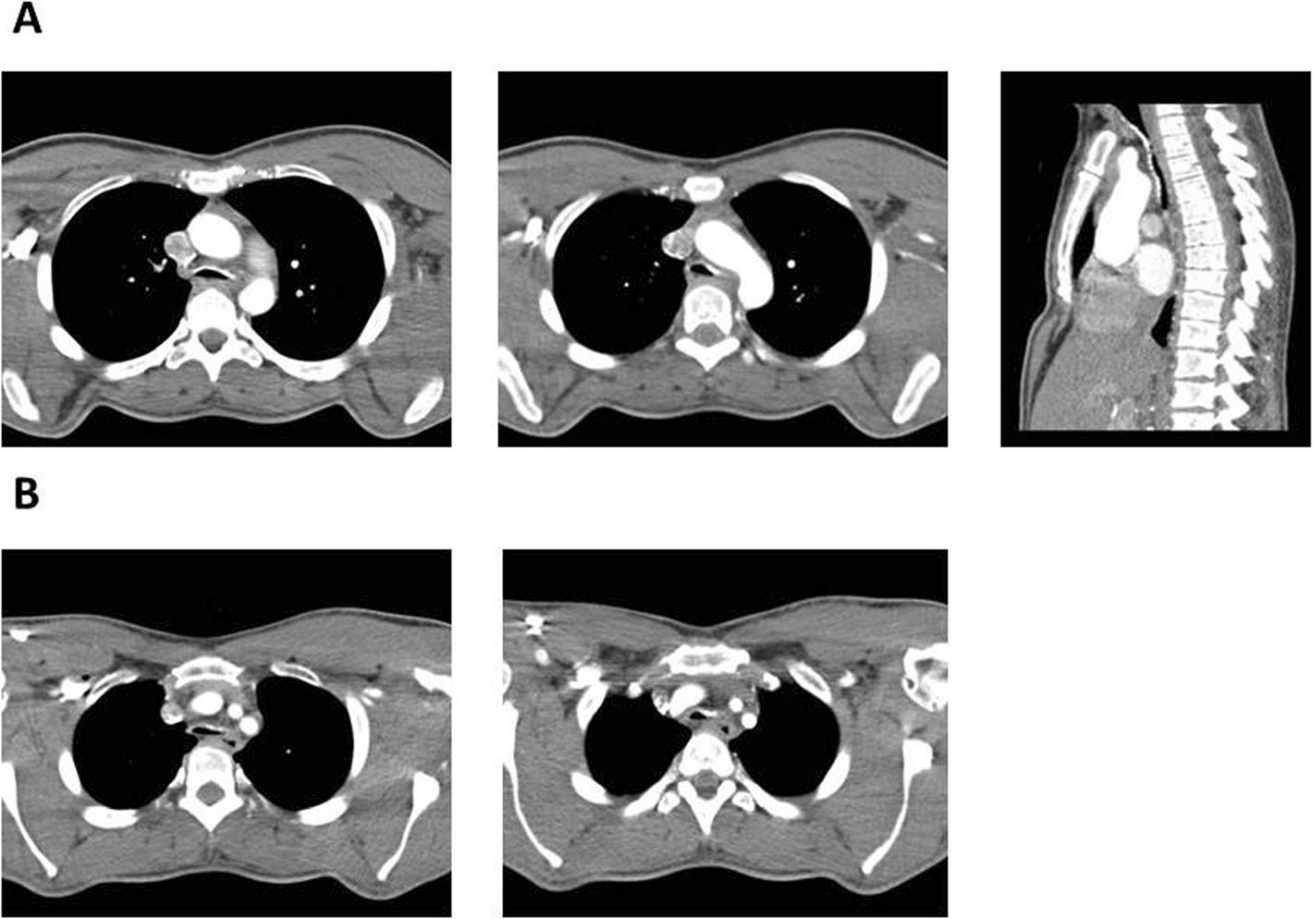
Computed tomography of the respiratory tract in MPSII patient with respiratory insufficiency. The computed tomography (CT) scan performed during the phonation and Valsalva test, revealed: an adynamic tracheobronchomalacia in the lower parts of the respiratory tract with a deformation and significant reduction of the respiratory pathway diameters. The deformed trachea had reduced sagittal dimension with a reduction of lumen in a distance of at least few centimetres, at its narrowest point to 0,4cm^2^, 4,5 × 9 mm. a) A significant reduction in the airway lumen observed in the MPS patient - multilevel tracheomalacia with excessive collapsibility of the trachea. b) Bronchomalacia with a significant, multilevel reduction in the peripheral airway lumen
